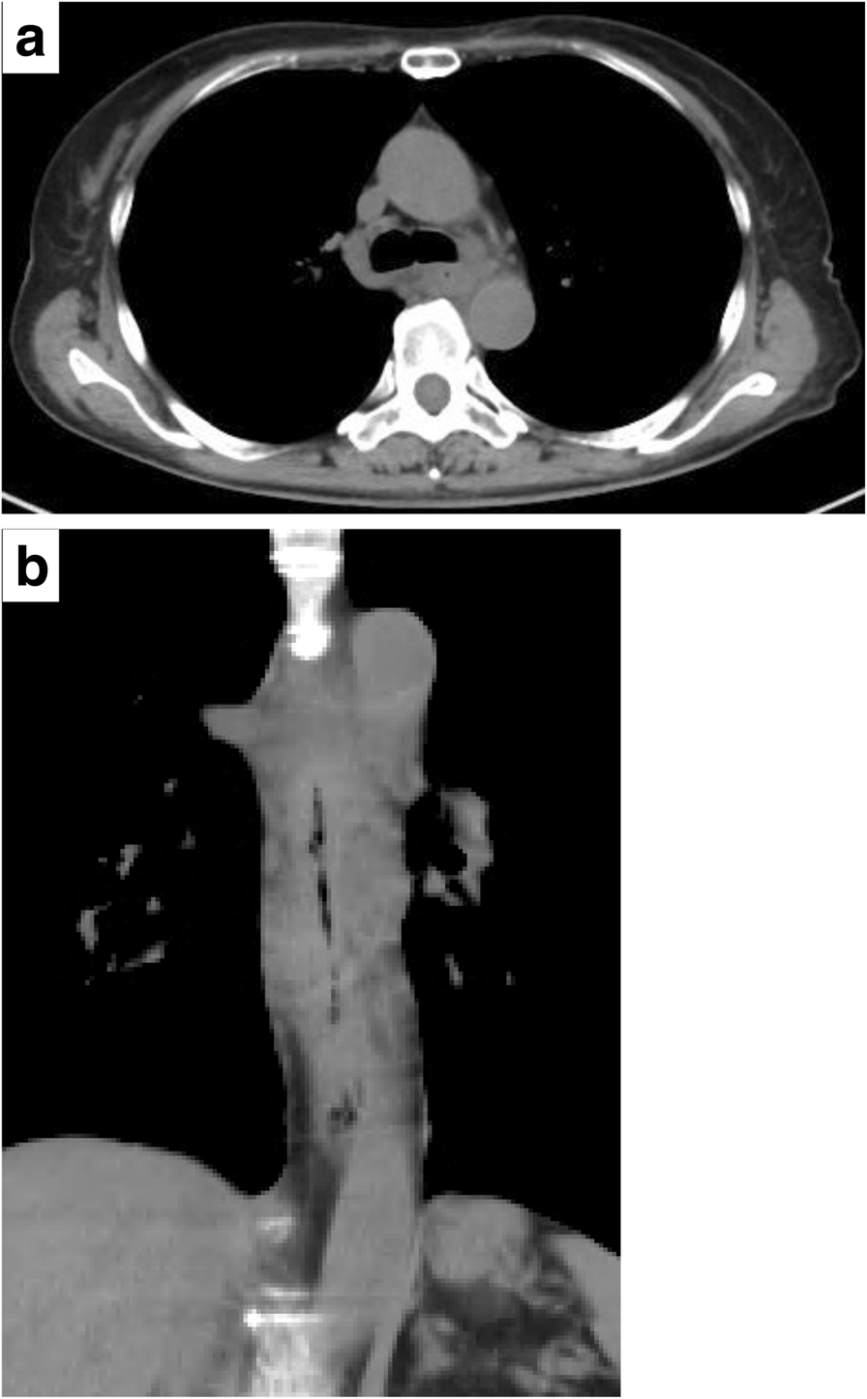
Preoperative CT. a Transverse image. b Coronal image: marked thickening of the esophageal wall was observed from the middle to lower thoracic esophagus
